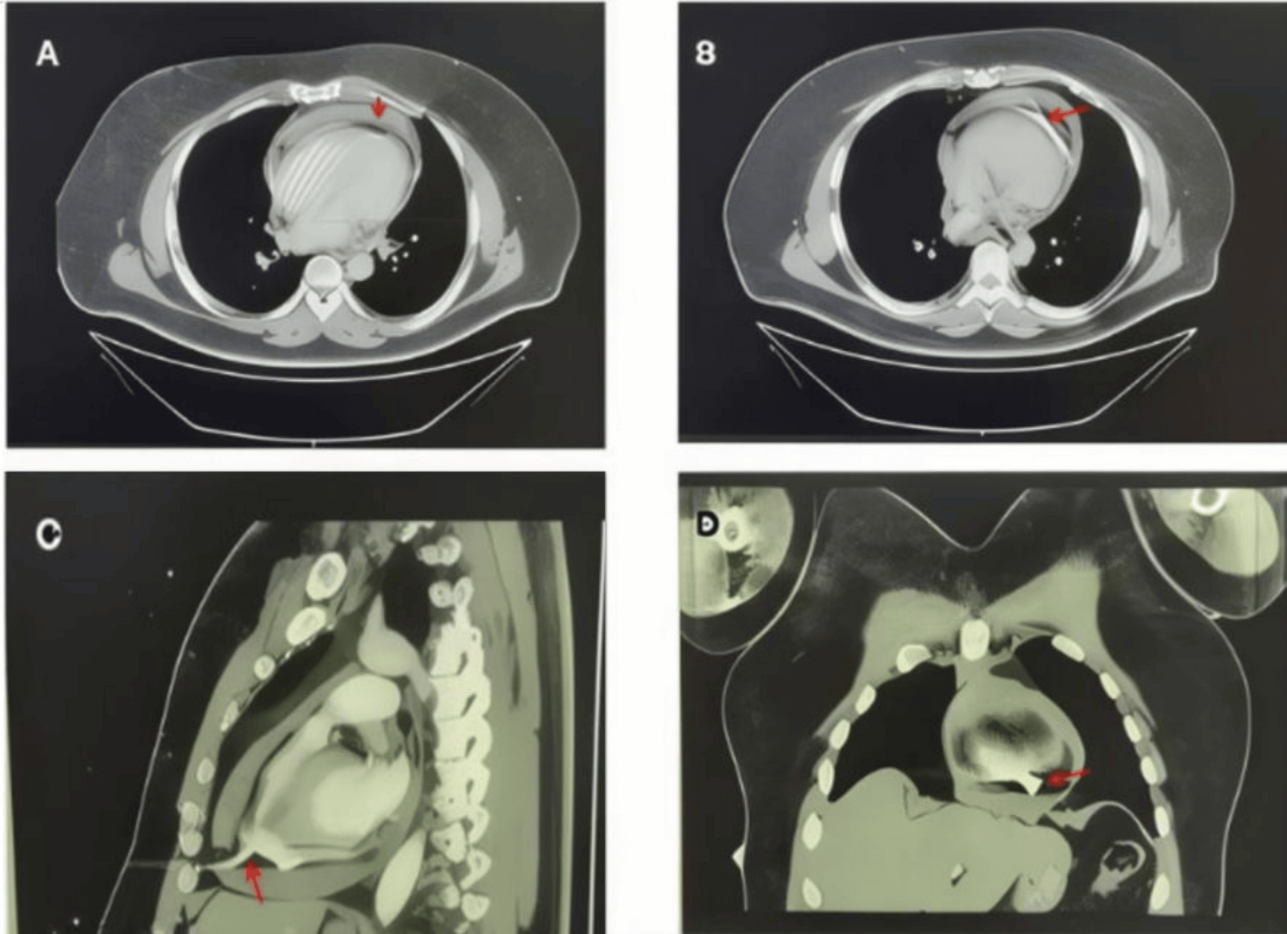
Computerized tomography (CT) of the chest brought from outside institution (A) and (B) are transverse showing pericardial effusion and retained bullet fragment, respectively, marked with red arrows. (C) is sagittal orientation showing a retained bullet fragment in the apical region marked by a red arrow. (D) is the coronal orientation scan showing the same findings. Red arrows used to mark pericardial effusion in A, bullet fragment in B, C, and D.
